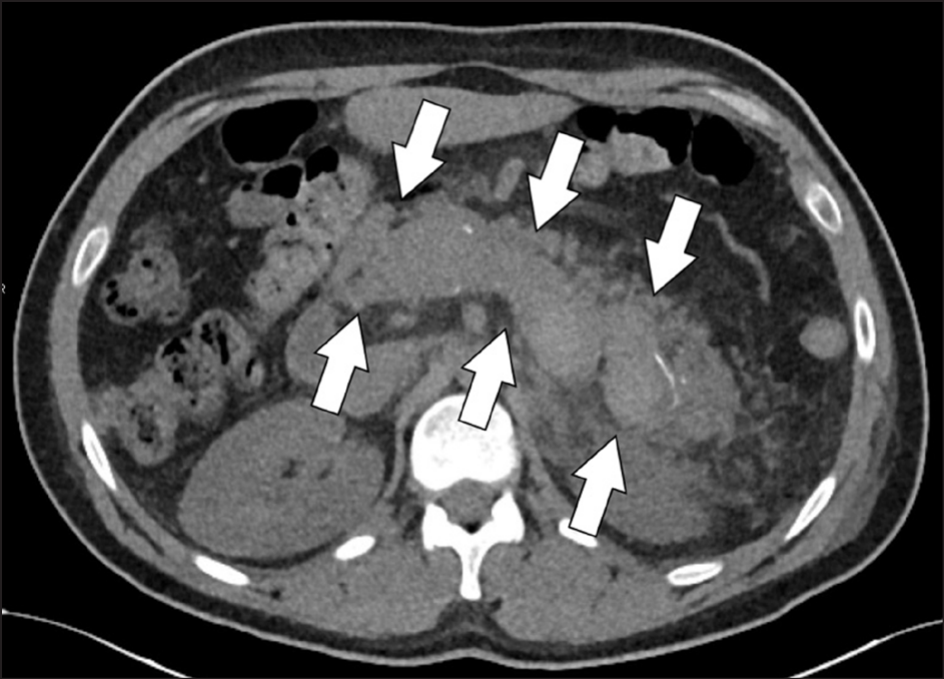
Axial unenhanced CT-slice revealing a spontaneously hyperdense splenic vein thrombus.
